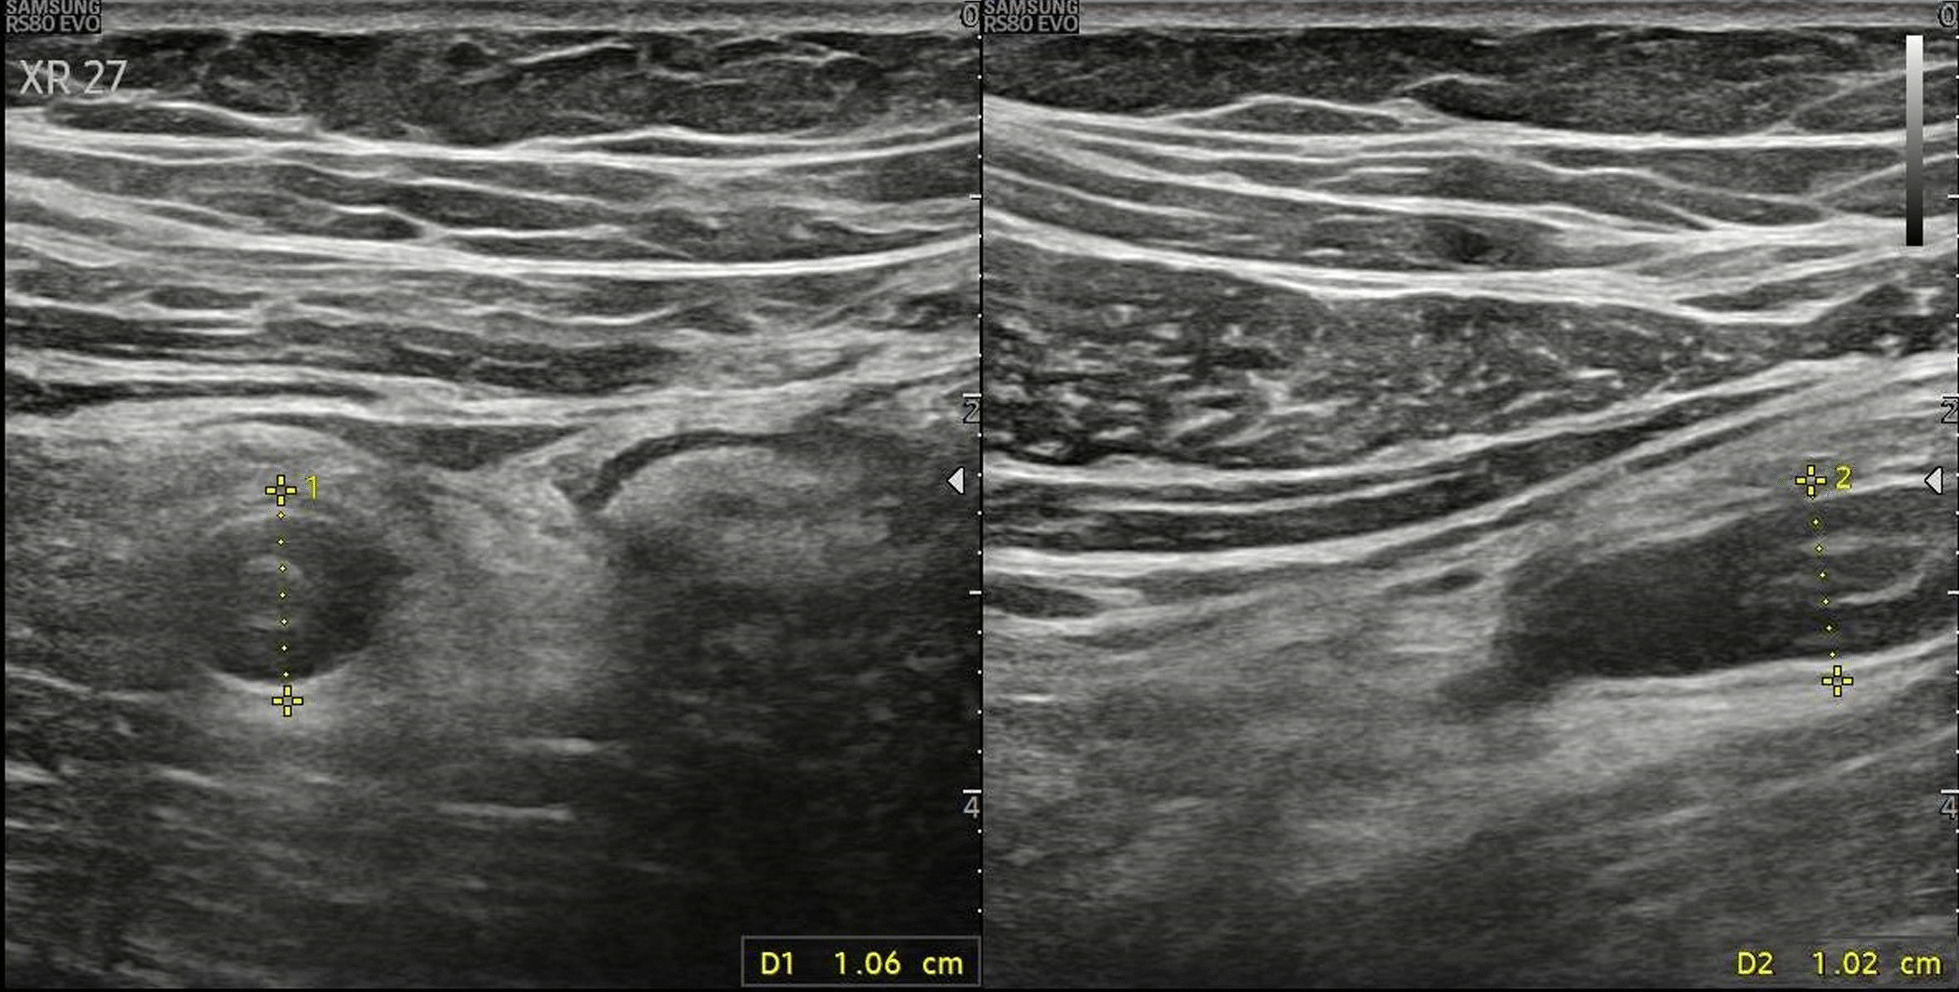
Abdominal ultrasonography showing a well-defined blind ended tubular non compressible structure seen at the upper limits of the right iliac fossa measuring about 3 cm in length and 1 cm in diameter
